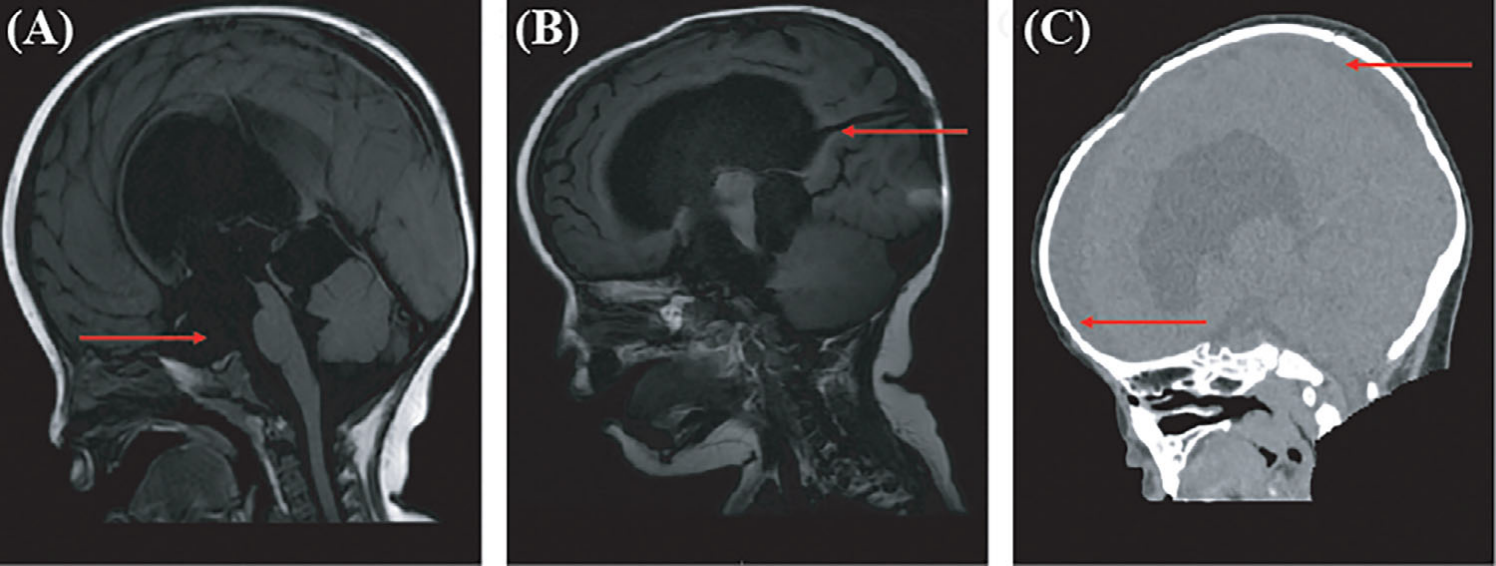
Image of patient 4. (A) Sagittal image magnetic resonance imaging (MRI) showing hydrocephalus prior to ventriculoperitoneal (VP) shunt with a small suprasellar cyst (red arrow); (B) Sagittal image MRI showing hydrocephalus after VP shunt with the ventricular canal shown with a red arrow; (C) After a neuroendoscopic third ventriculostomy and cyst fenestration, subdural effusion was observed in the prefrontal and parietal lobes (red arrow).
